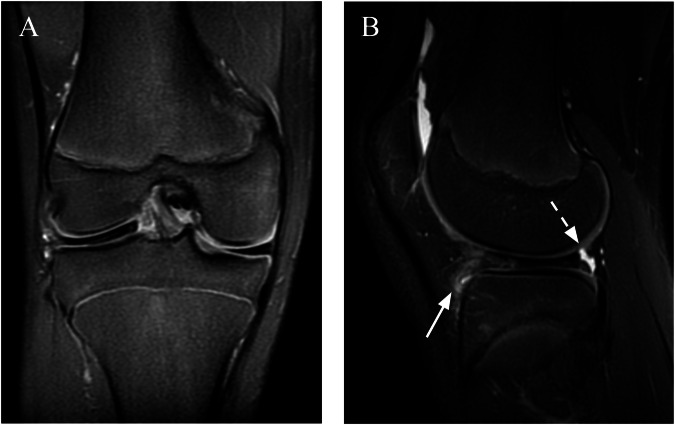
A 13-year-old boy complained of pain during running and leg extension movement. A Coronal and (B) sagittal FS T2-W MR images of the right knee show no shift-type complete lateral degenerated DM, parameniscal soft-tissue edema (arrow), and absence of the posterosuperior popliteomeniscal fascicle (dotted arrow). These are signs of PRI
